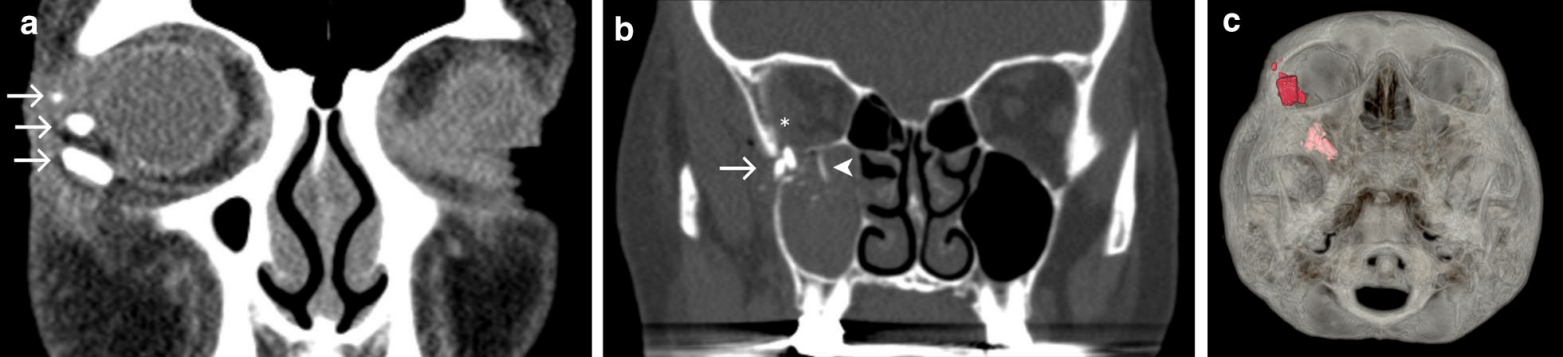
Broken water glass. Coronal CT sections (a, b) and 3-dimensional volume rendering (c) displaying intraorbital hyperdense foreign objects (arrows) in close proximity to the globe and the inferior oblique muscle (a) and inside the infratemporal fossa, protruding into the maxillary sinus and orbital cavity (b). Pronounced hyperdensity of the glass objects enables their differentiation from dislocated bone fragments (arrowhead). Also note intraorbital-subperiosteal haematoma (asterisk)
